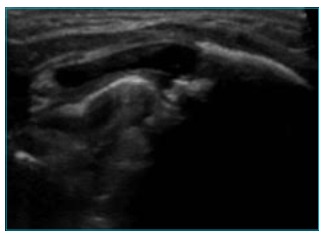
Ultrasound of left costal arch soft tissues of the. An irregularity
